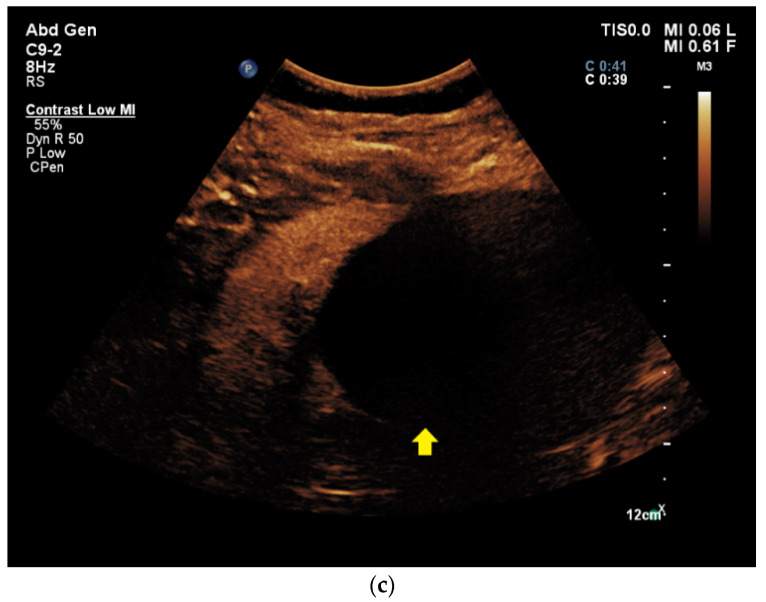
Contrast-enhanced ultrasound of a Bosniak II renal cyst. (a) In the native B-mode, a hypoechoic, homogeneous lesion with thin peripheral septa (yellow arrow) is detected in the right kidney. (b) The lesion shows no hypervascularization in Color Doppler (yellow arrow). (c) After the intravenous application of SonoVue^®^, no contrast-enhancement of the lesion can be visualized (yellow arrow).
